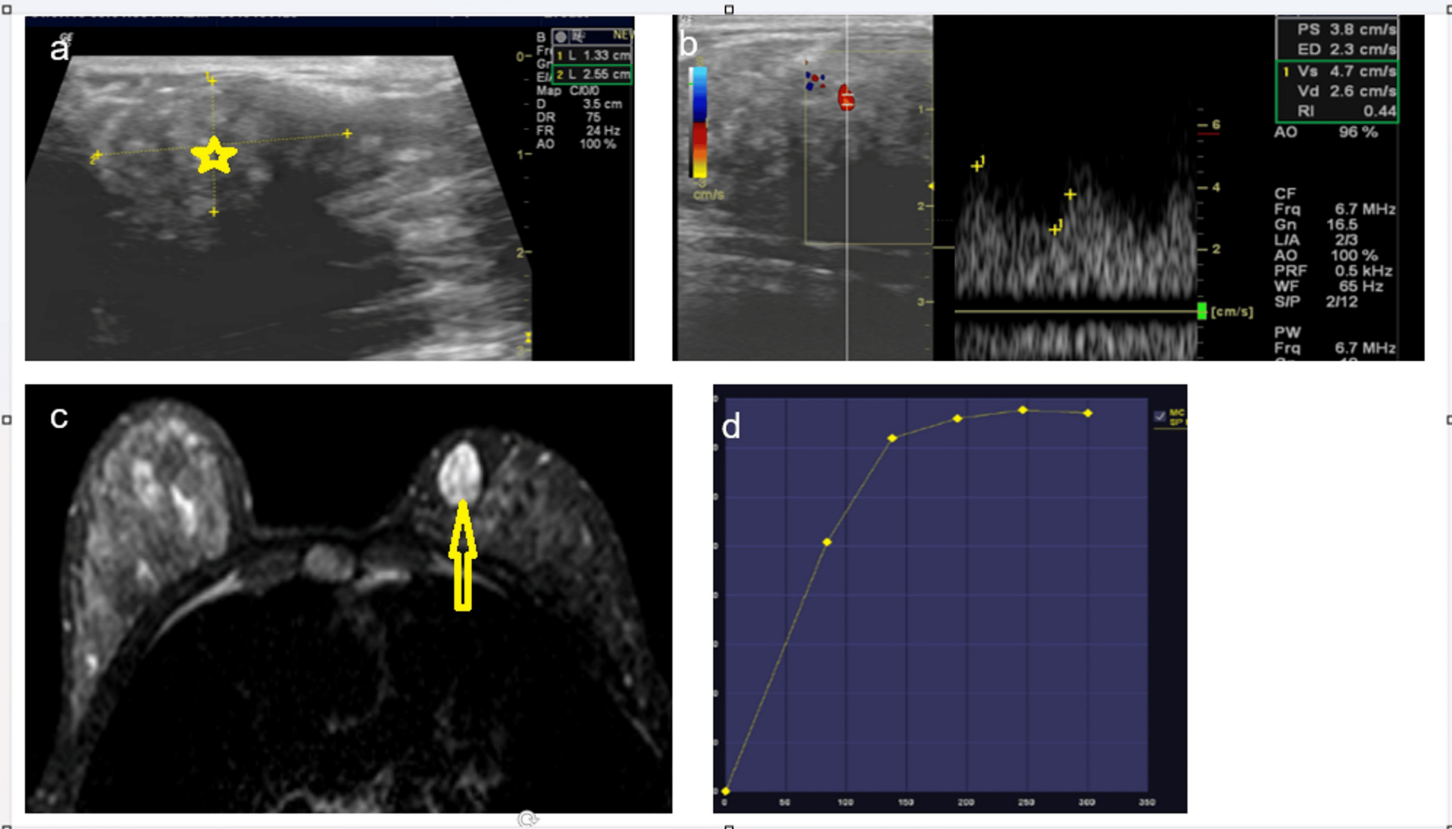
(A–D): Imaging features of fibroadenoma in a 20-year-old female involving the right breast (A) Gray-scale ultrasound shows a well-defined, oval, heterogeneous mass with circumscribed margins and parallel orientation (yellow star).
